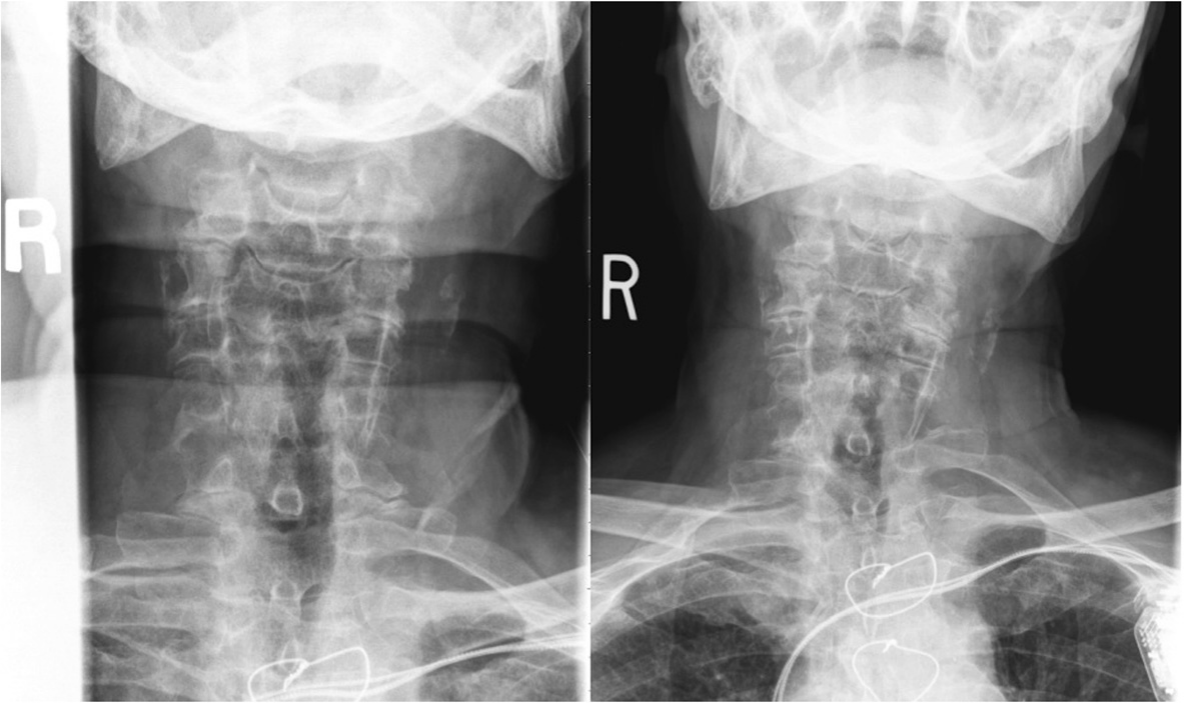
AP cervical radiograph taken in 2010 (*Left*) AP cervical radiograph demonstrating missing left C6 pedicle and articular pillar taken in 2014 (*Right*)
